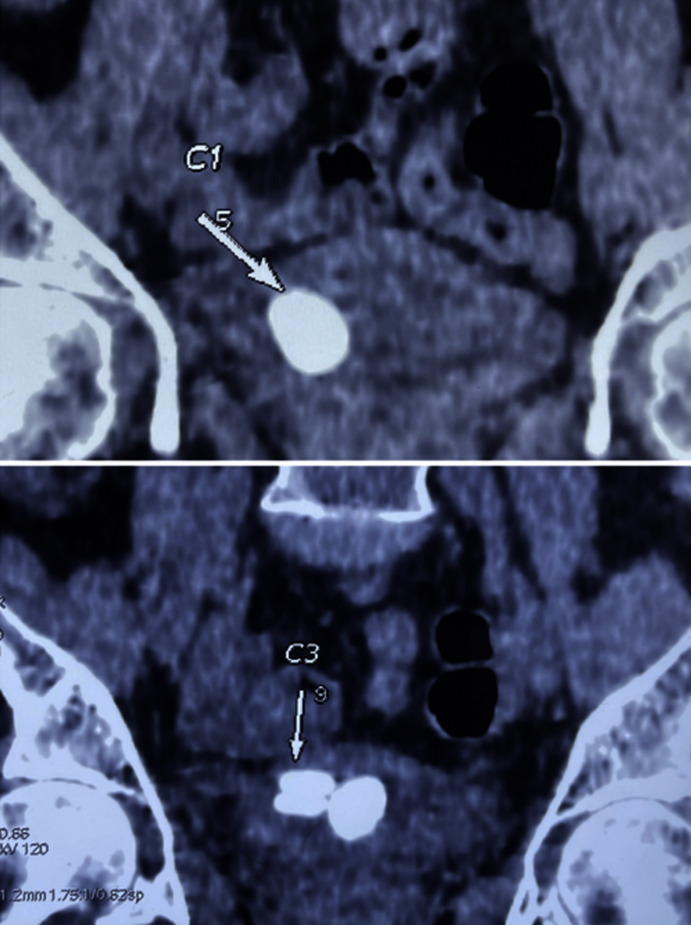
frontal section of a CT-Scan showing multiple neobladder stone
